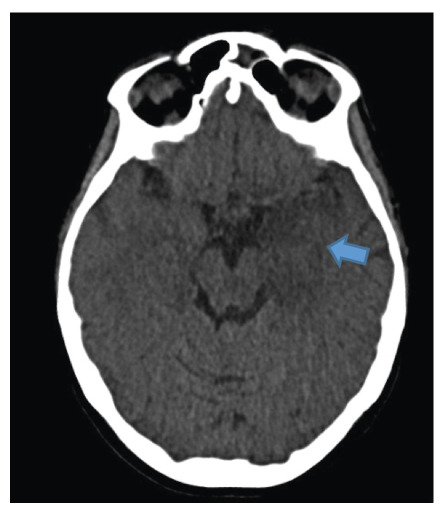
Initial CT scan of the patient. Hypodense lesion in left mesial temporal lobe with mass effect (blue arrow). Limbic encephalitis was suspected.
